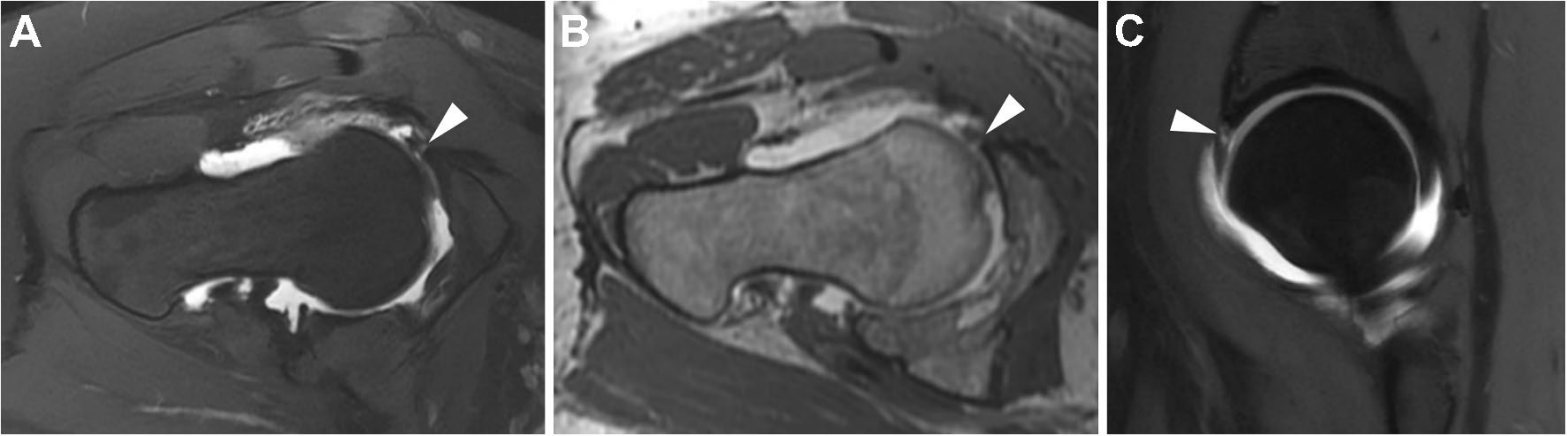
Postoperative hip imaging. Twenty-one-year-old woman with prior resections of cam and pincer lesions as well as a periacetabular osteotomy, presenting with hip pain. Axial oblique T2 fat-suppressed (A), radial T2-weighted (B), and sagittal T1-weighted fat-suppressed MR arthrogram images show a surgically confirmed recurrent tear of the labrum extending from 10 o’clock to 2 o’clock (arrowheads)
